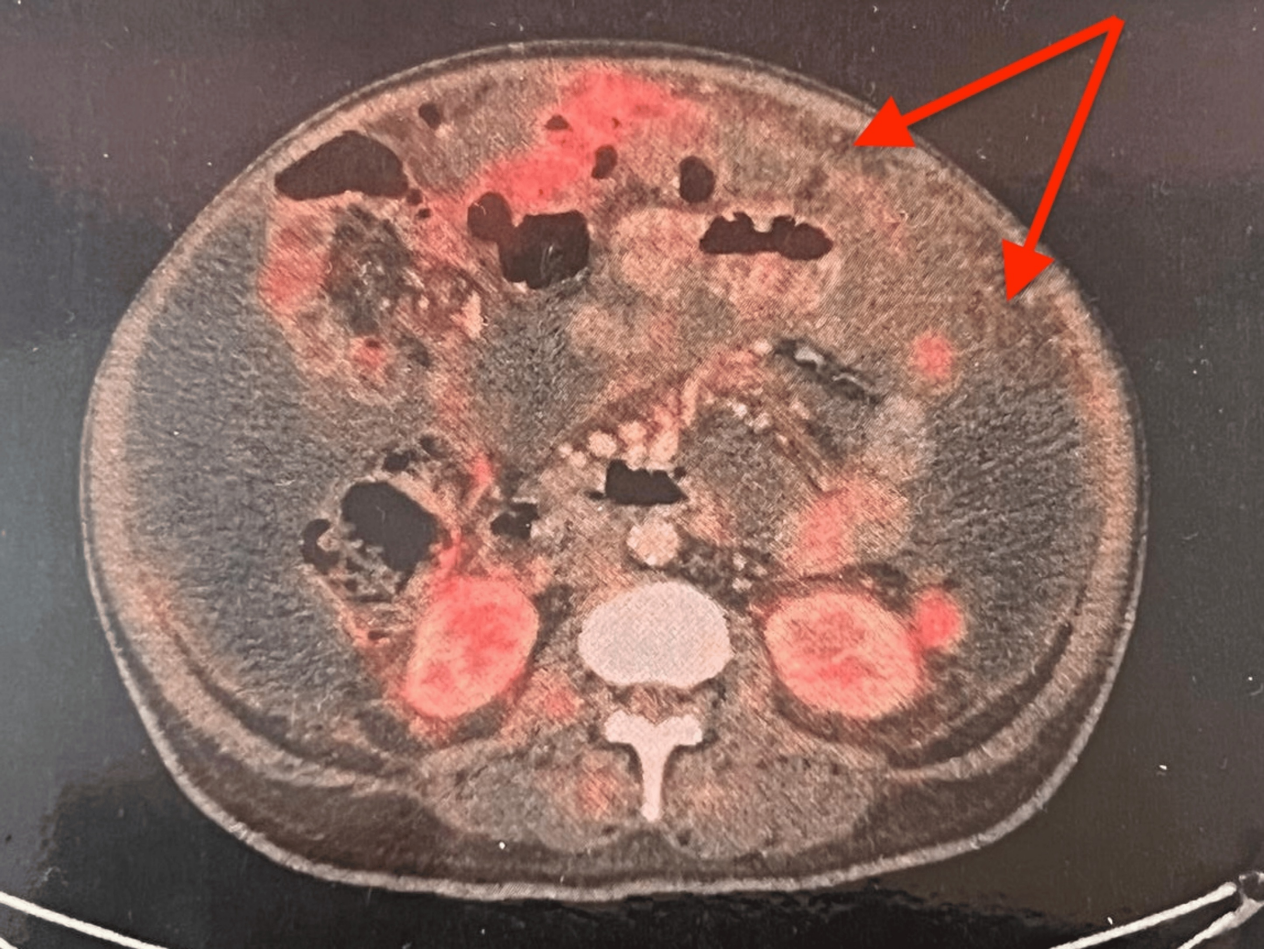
Low-grade FDG uptake with omental stranding and ascites FDG: fluorodeoxyglucose
